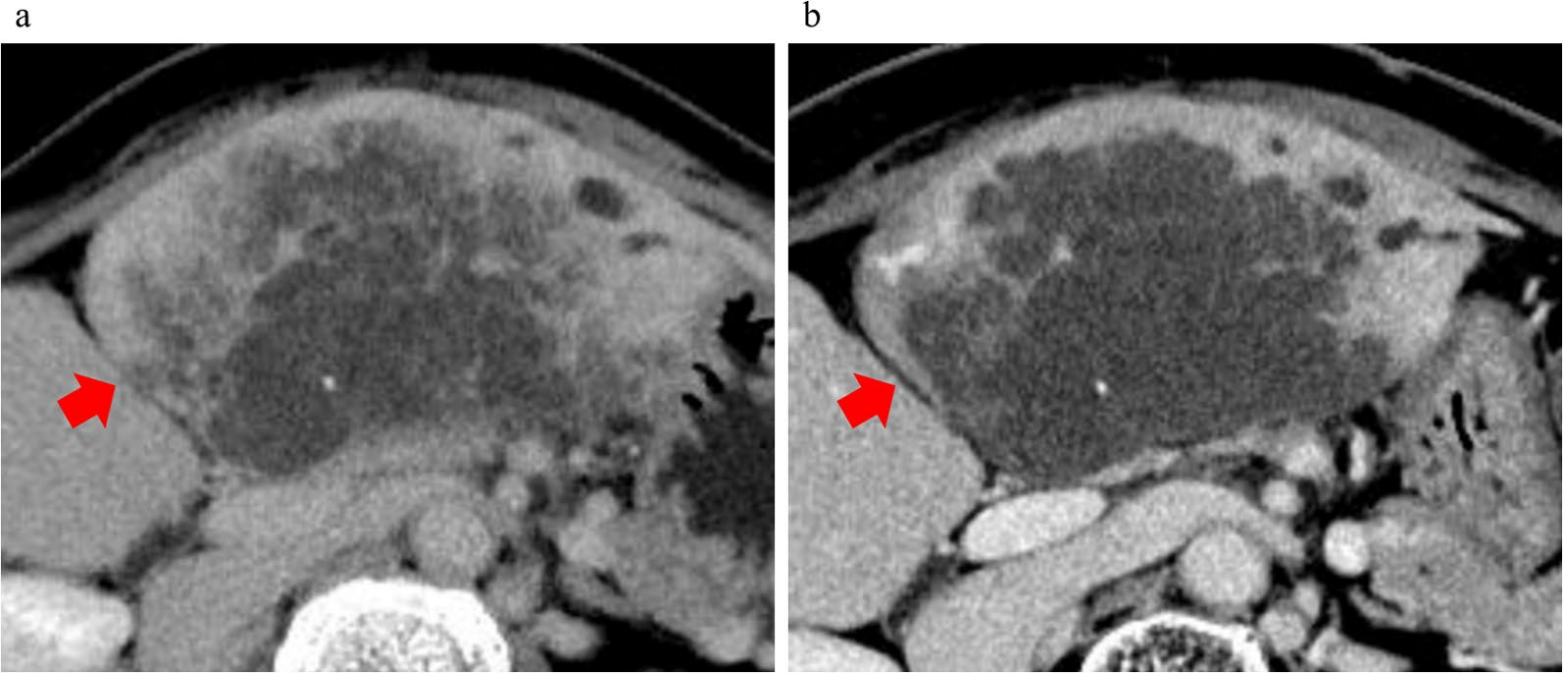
a Image findings of contrast-enhanced dynamic-CT before neoadjuvant chemotherapy (NAC) of Case 1. Image showed a 11 × 7.5 cm cystic lesion in the lateral segment of the liver with a faint ring-enhancement (red arrow). b Image findings of contrast-enhanced dynamic-CT after NAC of Case 1. The shrinkage of the tumor was not observed (red arrow). Response Evaluation Criteria in Solid Tumors (RECIST) is stable disease
